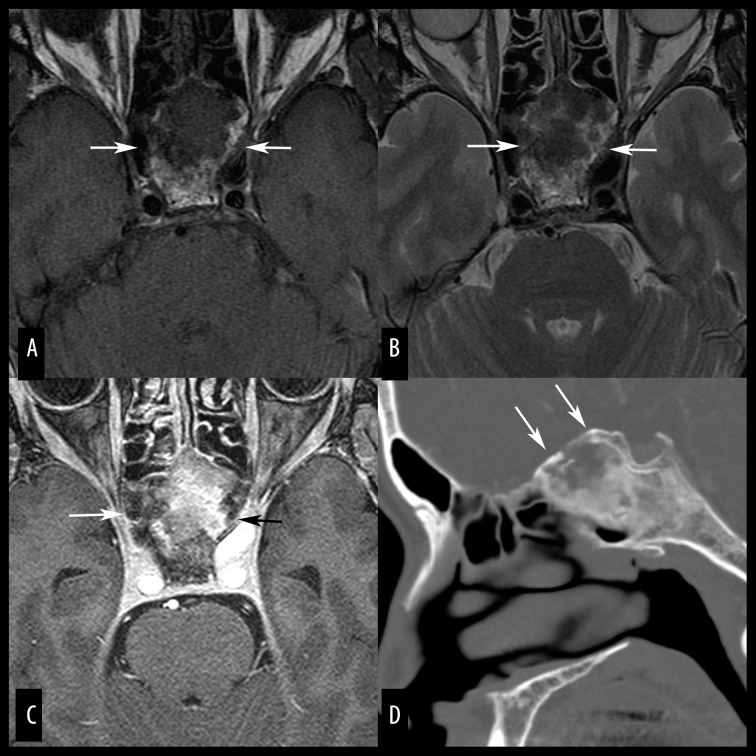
Fibrous dysplasia of the sphenoid in a 61-year-old man. (A, B) Axial, T1-weighted (A) and T2-weighted (B) MR images show a mass lesion (arrows) of the sphenoid with mixed intensity ranging from hypo- to hyperintense. (C) Axial, fat-suppressed, contrast-enhanced MR image reveals inhomogeneous but strong enhancement of the mass (arrows). (D) Sagittal-reformatted bone window CT image shows a mixed ground-glass and cystic appearance that is typical of fibrous dysplasia. A convex margin (arrows) also suggests fibrous dysplasia rather than arrested pneumatization. Fibrous dysplasia incidentally found in the elderly can be mistaken for a neoplasm on MR images.
